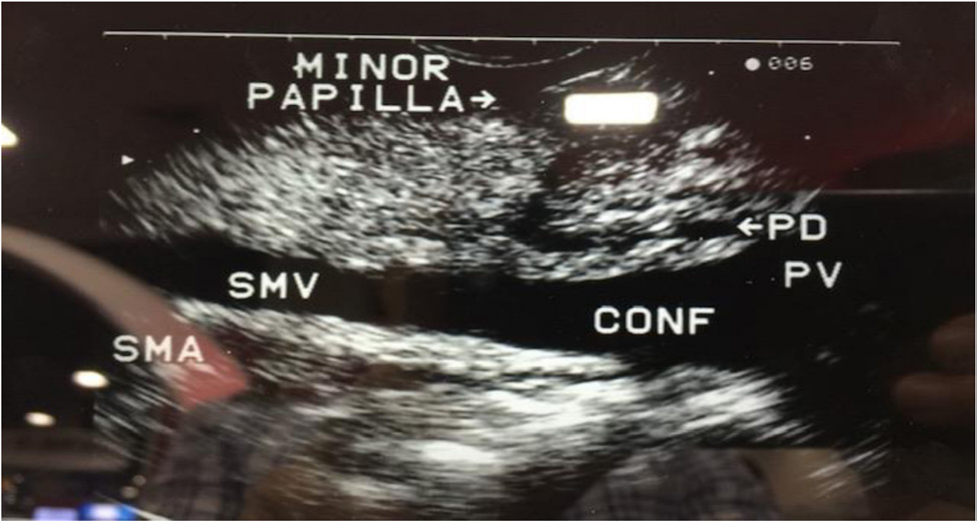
Fourteen-year-old with recurrent pancreatitis. EUS showed the PD connecting with the minor papilla diagnosis pancreas divisum. EUS = endoscopic ultrasound; PD = pancreatic duct.
